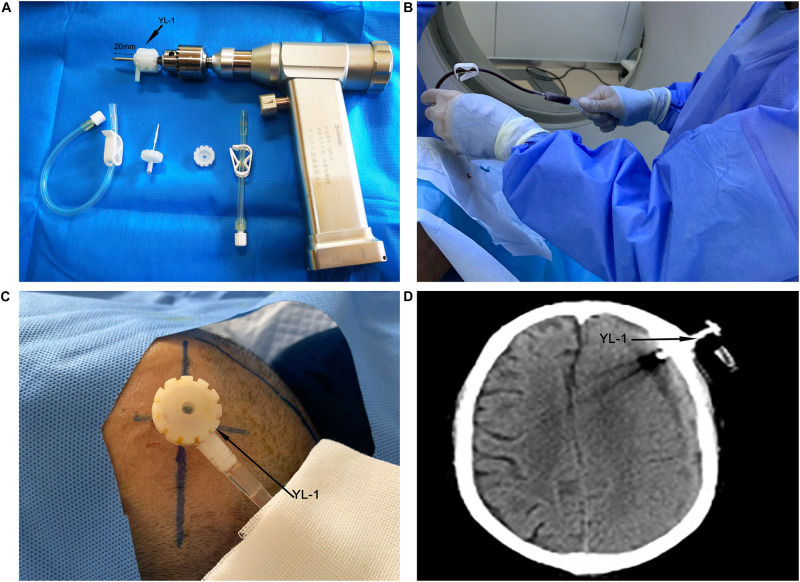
Yl-1 drainage tube (black arrow) before surgery, drill length 20 mm (two black arrows) (A). YL-1 drainage tube was used to draw and rinse the CSDH during the operation (B). YL-1 drainage tube (black arrow) continued to drain the hematoma after the operation (C). YL-1 drainage tube (black arrow) on postoperative CT (D).
